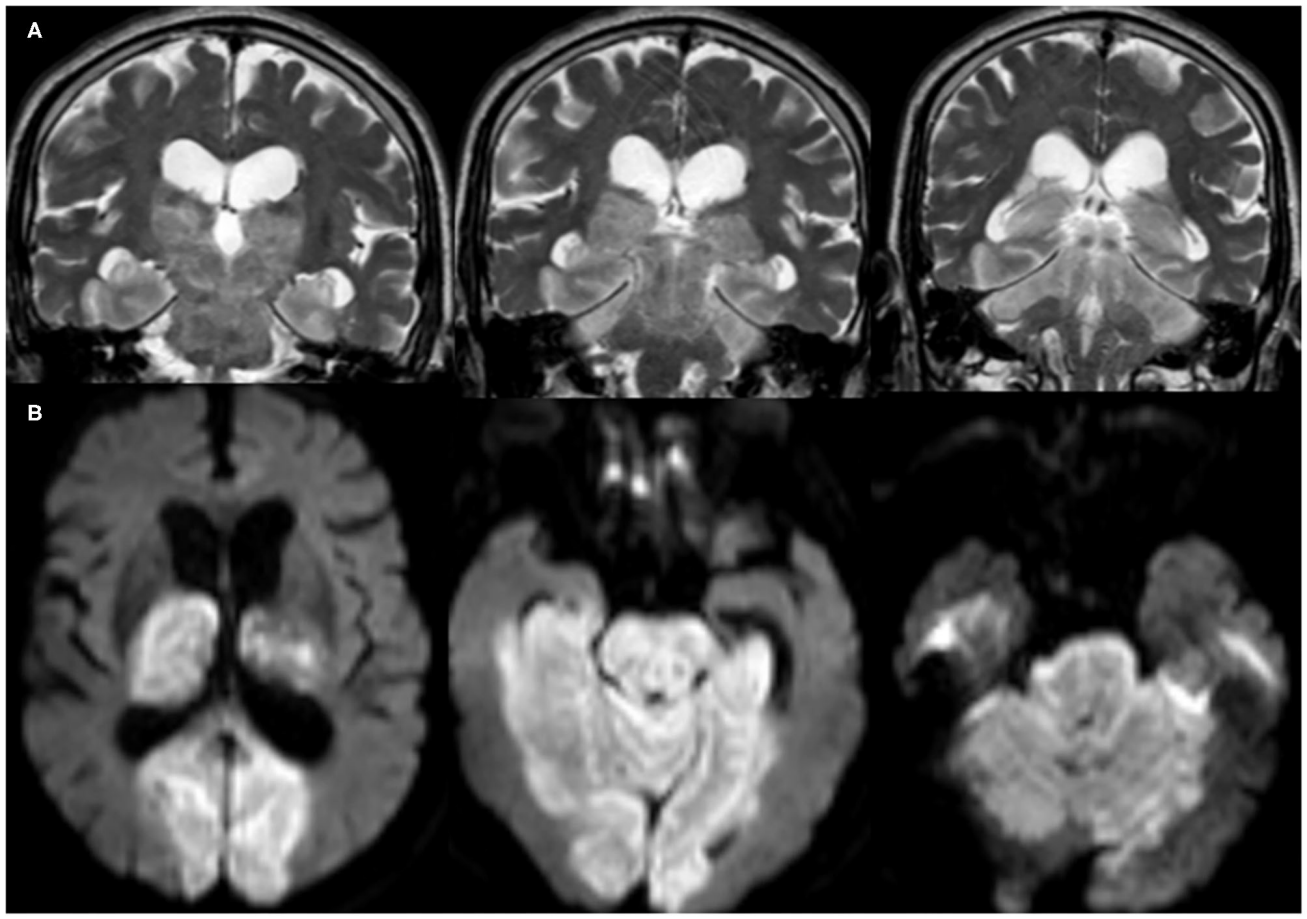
Brain MRI, (A) coronal T2-weighted imaging (T2WI), and (B) axial diffusion-weighted imaging (DWI) of a 76-year-old male patient show acute infarct (large vessel occlusion) involving the basilar artery territories (brain stem, bilateral thalami, occipital and inferior temporal lobes, as well as the cerebellum).
